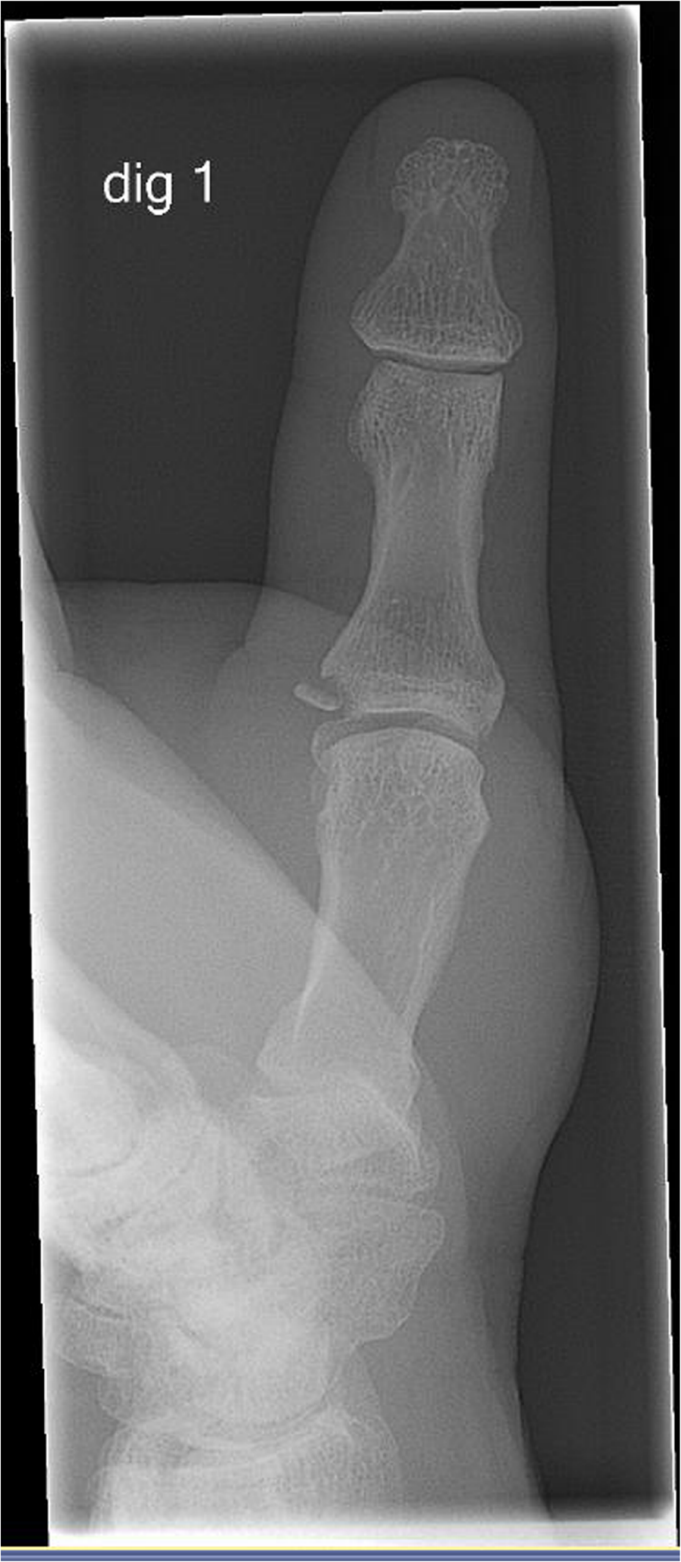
Radiograph of a bony skier’s thumb.
